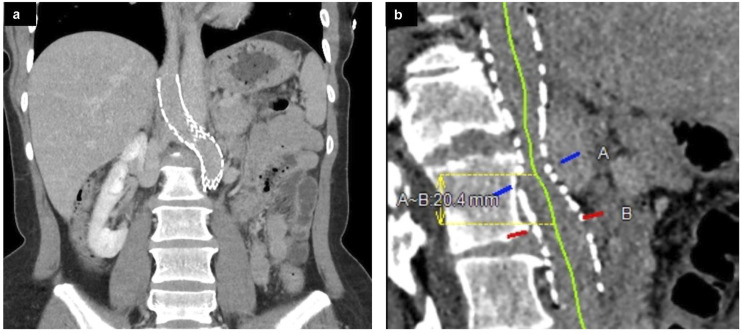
(A) Contrast-enhanced abdominal CT with coronal reformatted images obtained 2 months post-procedure demonstrates the course of the stent. (B) Curved multiplanar reformatted images demonstrate mild compression of the stented LIVC/renal vein behind the aorta with persistent patency.
